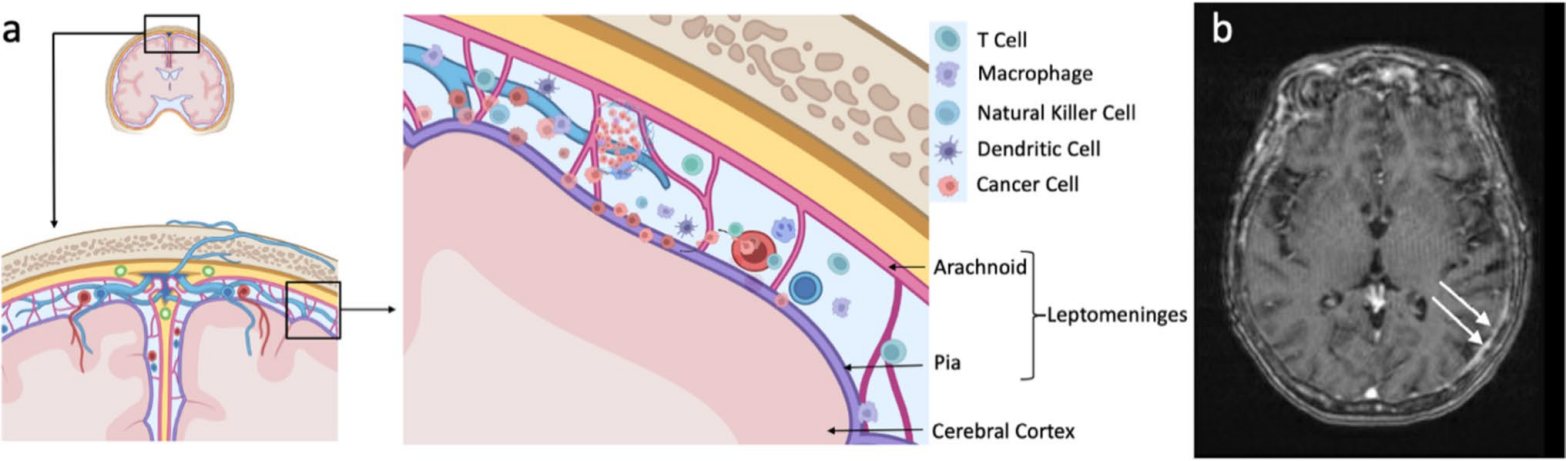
a Representation of LMD microenvironment with immune infiltration. b MRI of the brain of a patient with LMD from human epidermal growth factor-2 (HER2) positive breast cancer. Dashed arrows indicate leptomeningeal enhancement consistent with LMD (Image created using BioRender.com)
